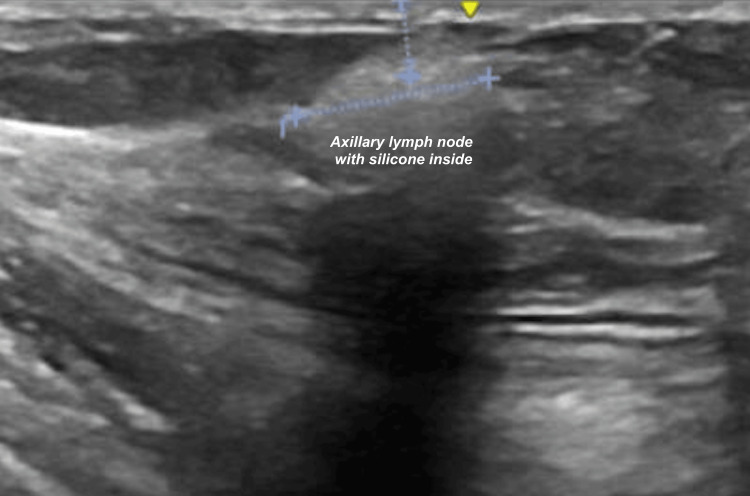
Ultrasound of the right axilla showing lymph nodes containing hyperechoic material consistent with silicone deposition
